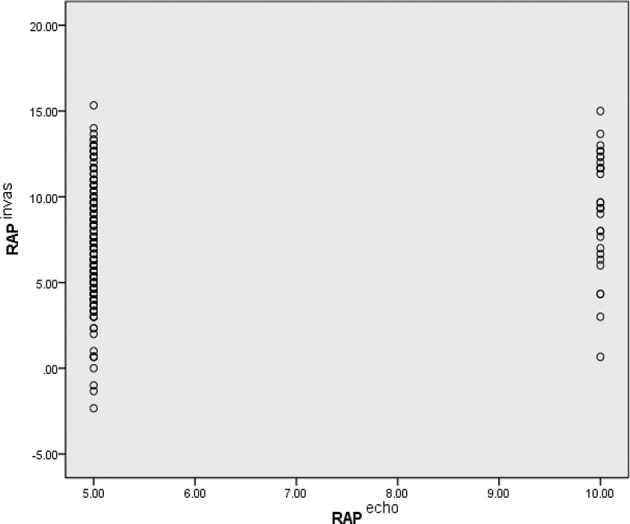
Comparison of RAP as estimated by D-ECHO and RHC. Echo, measured by Doppler echocardiographic; invas, invasive measurement by RHC; RAP, right atrial pressure.
